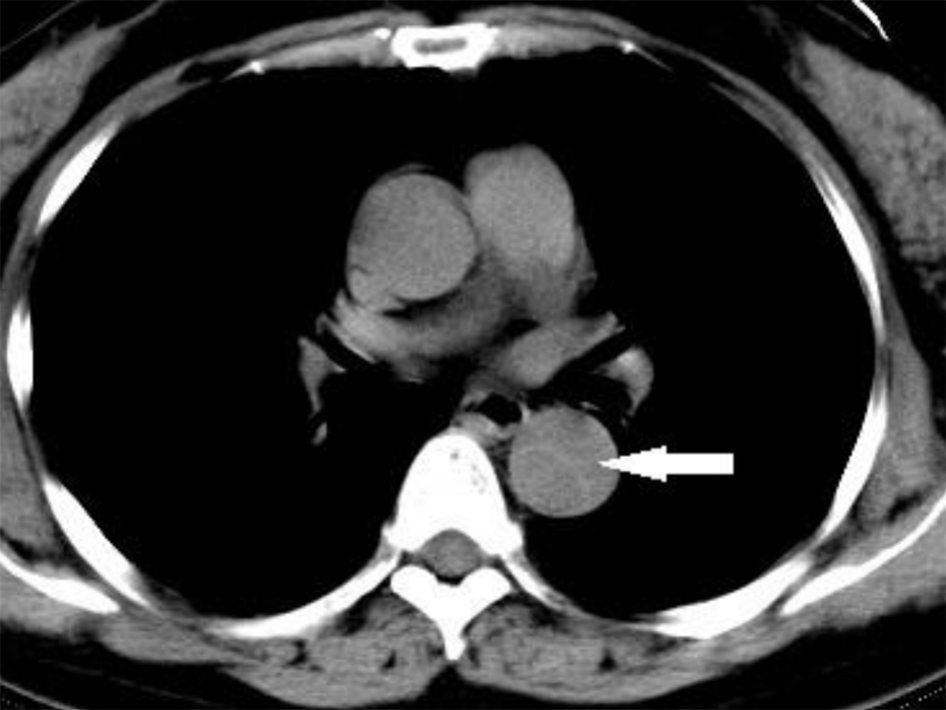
AAD found by chest CT; the arrow points to the inner wall of aortic blood vessel.
